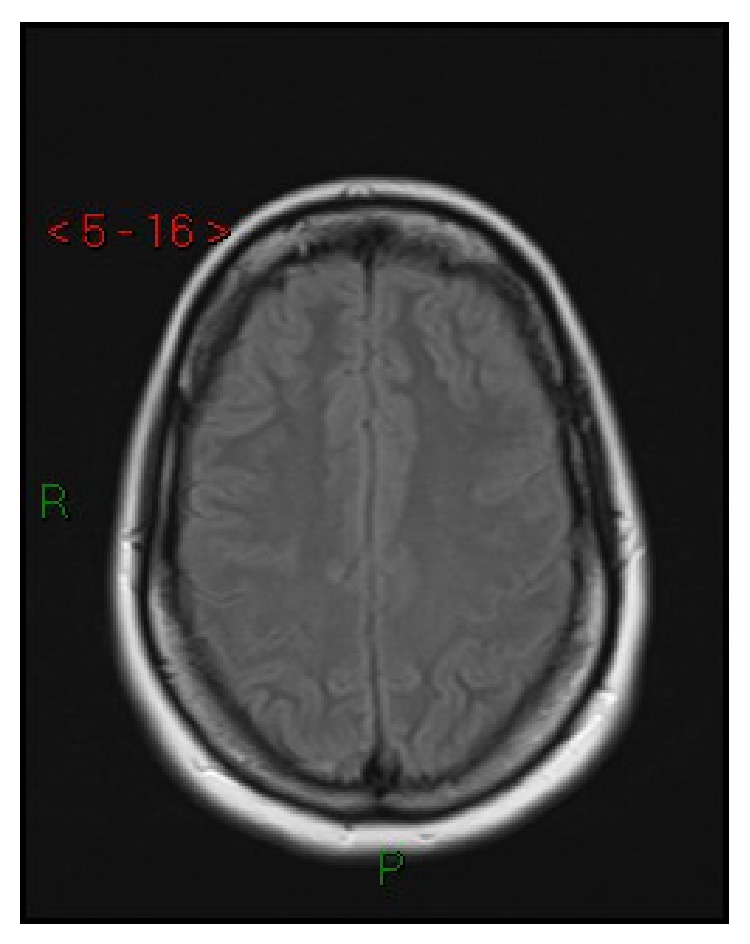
Case 2. Axial T2-FLAIR MRI: absence of lesions after 7 days.
